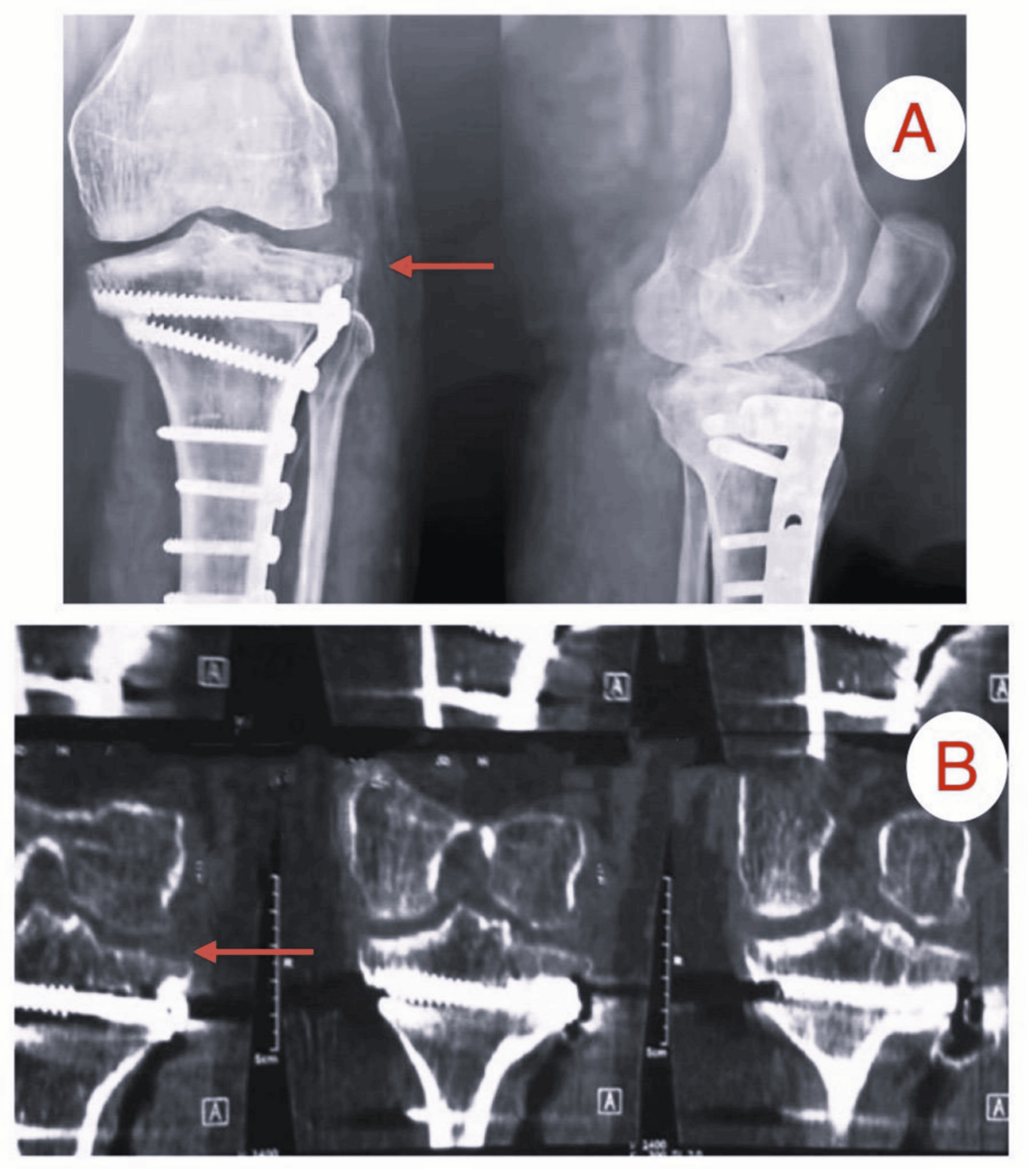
Immediate postoperative images of Patient A: (A) X-ray showing reduction of depressed plateau fracture, (B) CT scan showing an elevated reduced plateau fracture
